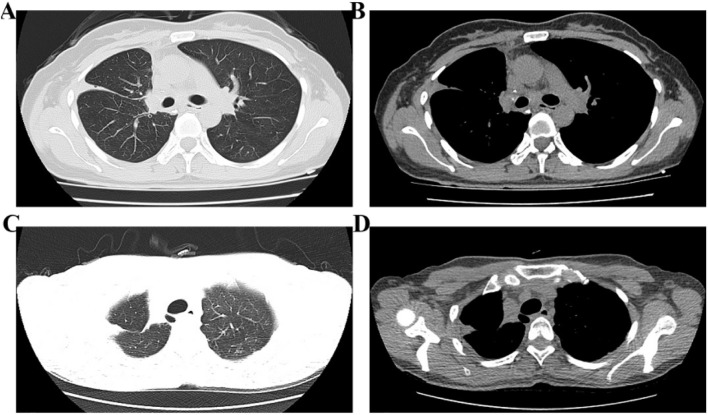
The results of the patient’s chest CT plain scan before discharge and during the follow-up outside the hospital. (A,B) Before discharge; (C,D) After discharge.
